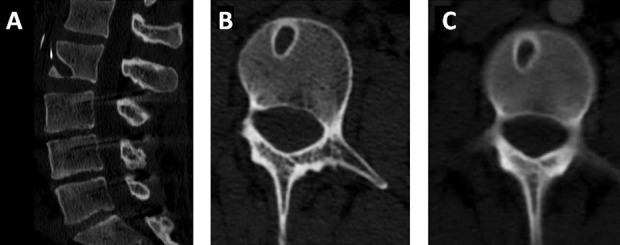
Erosive changes of the L2 vertebral body CT imaging demonstrates erosive changes of the L2 vertebral body with a prong of the IVCF violating the outer cortex (Figure 1A).
